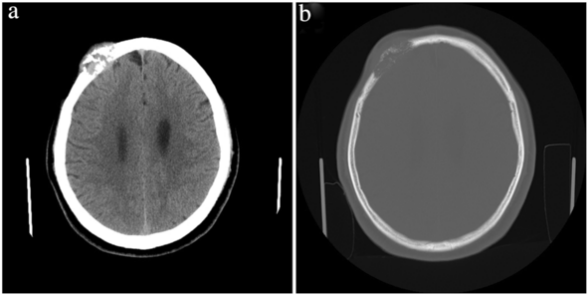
Preoperative CT scan of the patient. The lesion locates in the right frontal bone, isointense on CT scan, with prominent bone destruction. (a) CT scan of the soft tissue. (b) CT scan of the cranial bone.
